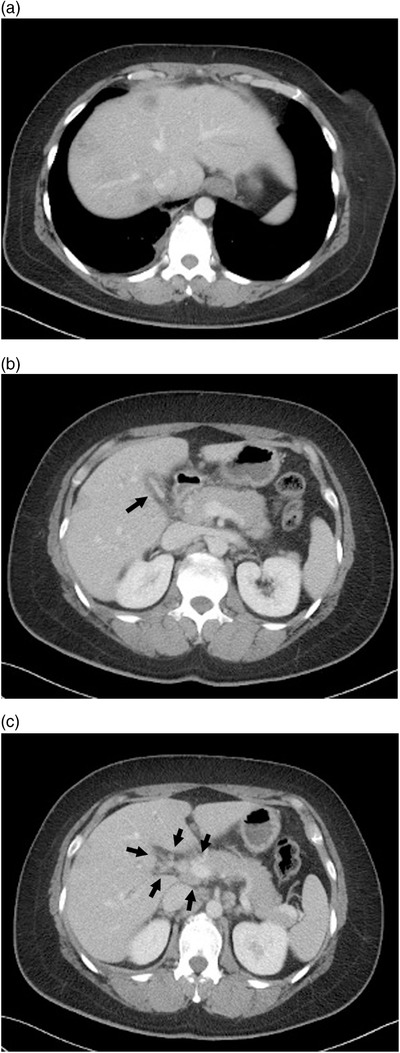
Contrast‐enhanced computed tomography. (a) Multiple intrahepatic low‐contrast density masses. (b) The gallbladder was shrunk and edematous with a thickened wall (arrow). (c) Enlarged hilar lymph nodes of the liver (arrow)
